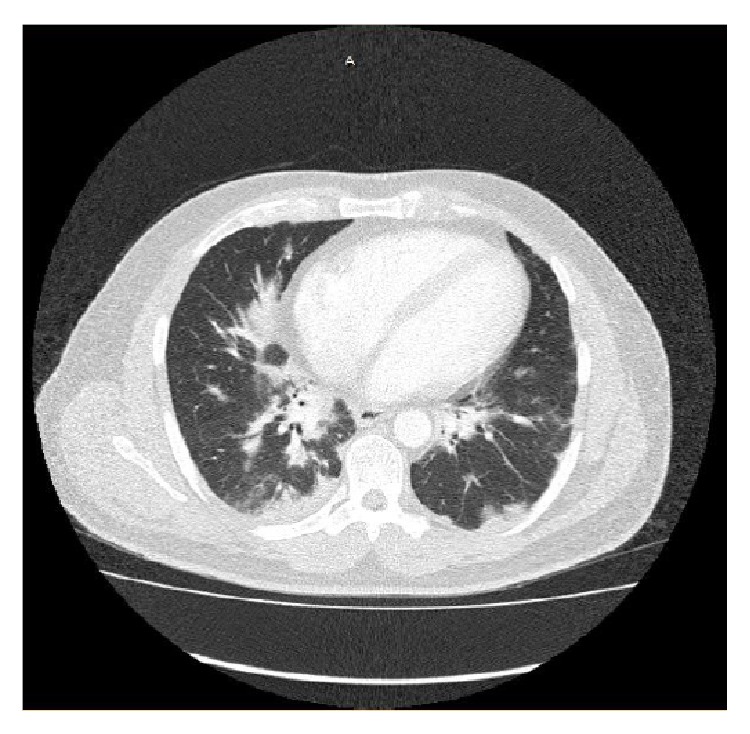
CT chest revealing bilateral basilar fibrosis along with reticulonodular and subpleural wedge shaped opacities.
